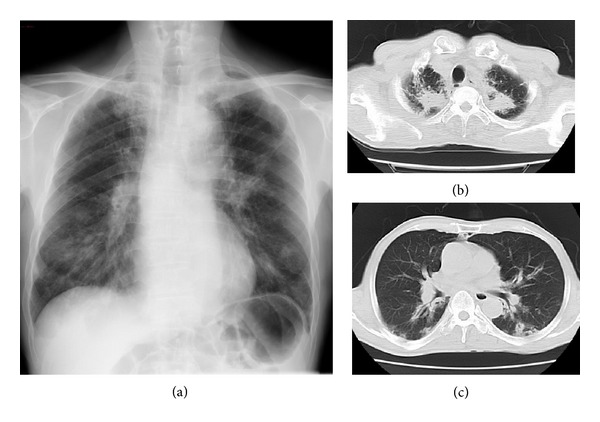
(a) Chest X-ray showed multiple small infiltrates in both lungs. ((b), (c)) Chest CT showed multiple nodules in the apex and the remarkable bronchial wall thickening in lower areas of both lungs.
